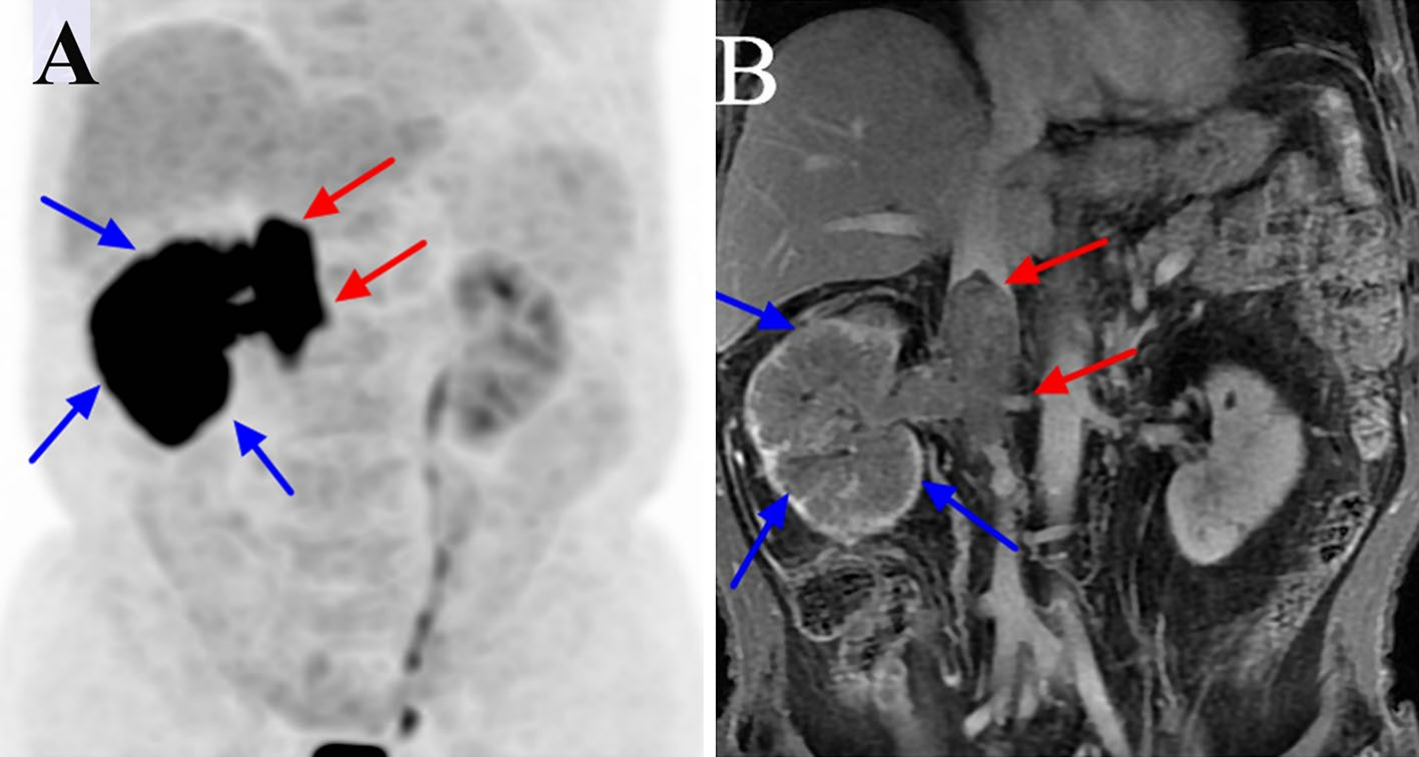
^18^F-FDG PET/CT and contrast-enhanced MRI (CEMRI) scans of a 77-year-old man with papillary cell carcinoma of the right kidney associated with a level II venous tumour thrombus (VTT). The VTT had invaded the venous wall and was graded as level II by PET/CT and CEMRI. The site of diffusely increased FDG uptake (SUVmax 9.6) on the PET maximum intensity projection image is the primary tumour (blue arrow) (a). The site of pathologically increased FDG uptake (SUVmax 12.5) from the right renal vein to the inferior vena cava is the VTT (red arrow) (a). The VTT had a regular shape, was enhanced and completely occluded the inferior vena cava lumen on coronal CEMRI (red arrow) (b), and the primary tumour showed diffuse heterogeneous enhancement (blue arrow) (b).
